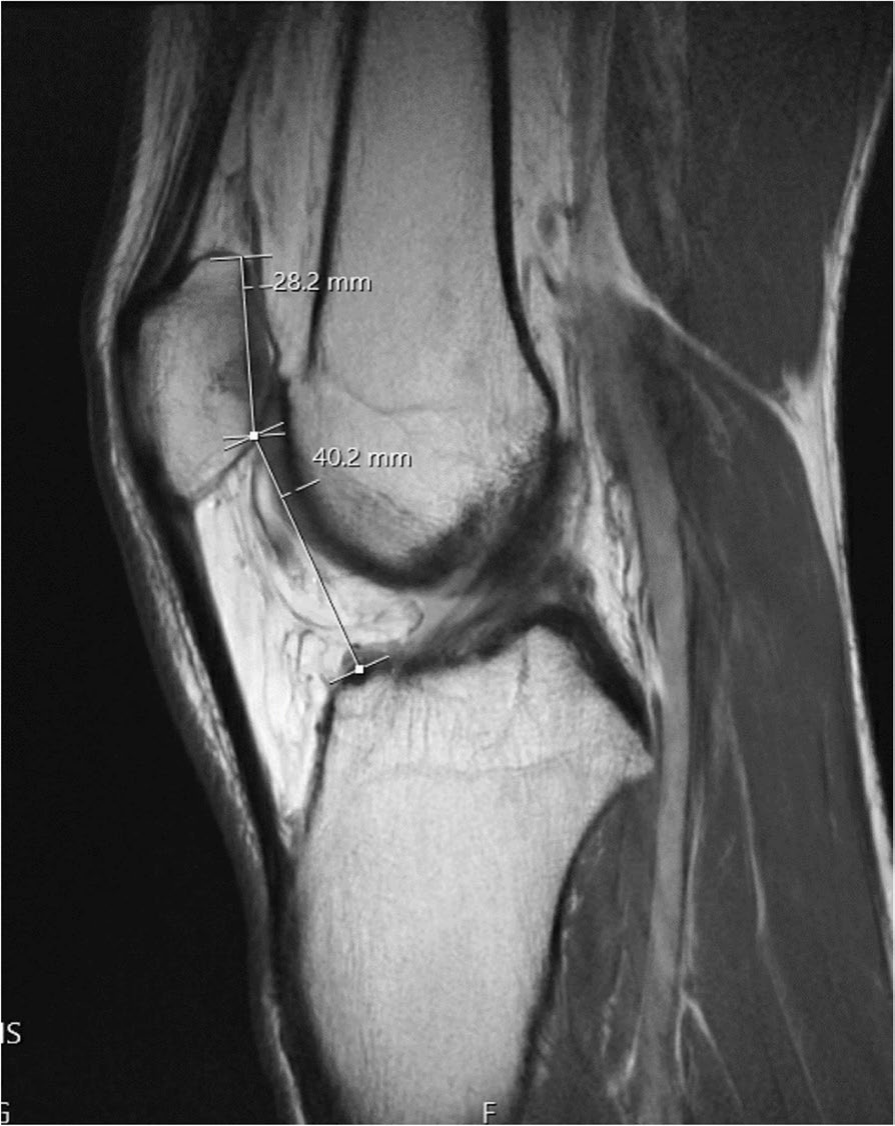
Caton Deschamps Index calculated on sagittal MRI as the ratio of the distance between the articular surface and the inferior aspect of the articular surface of the patella divided by the length of the articular surface of the patella
